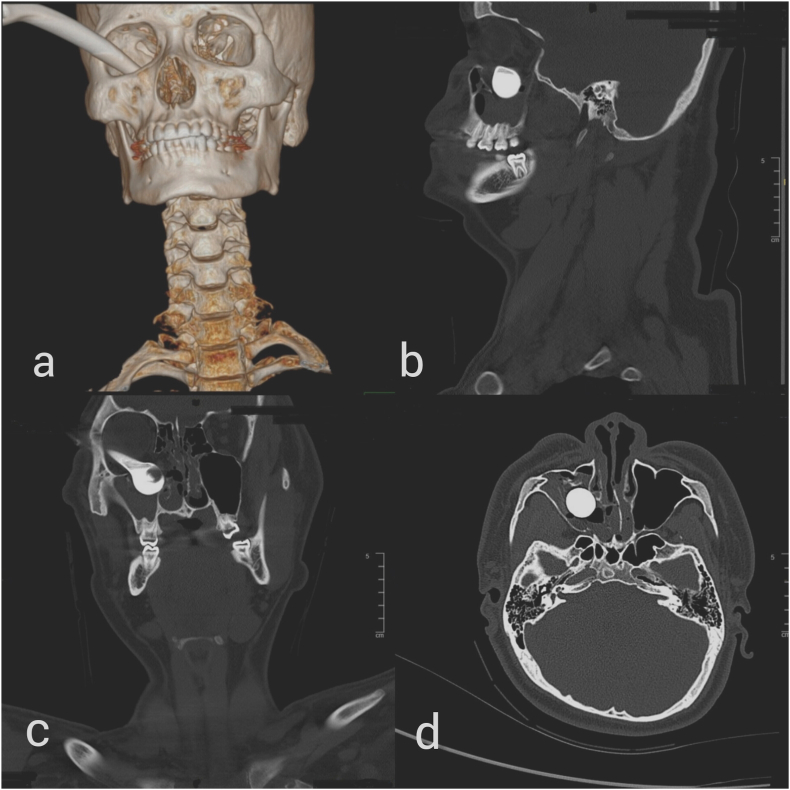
(a) CT imaging with 3D reconstruction showing entry of brake lever into orbit. CT imaging in (b) sagittal cut, (c) coronal cut and (d) axial cut showing the bulbous tip of the brake within the maxillary sinus.
